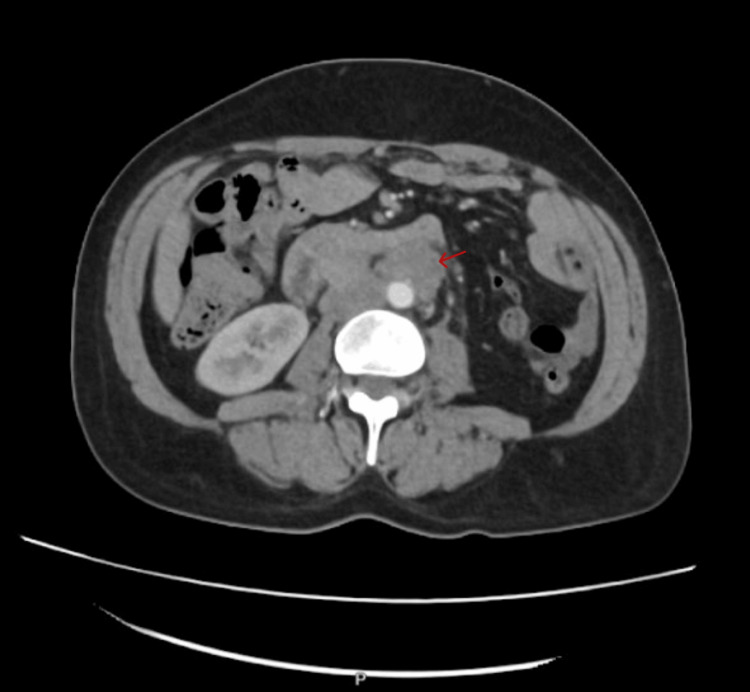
CT abdomen and pelvis axial view shows an enlarged right internal iliac lymph node
